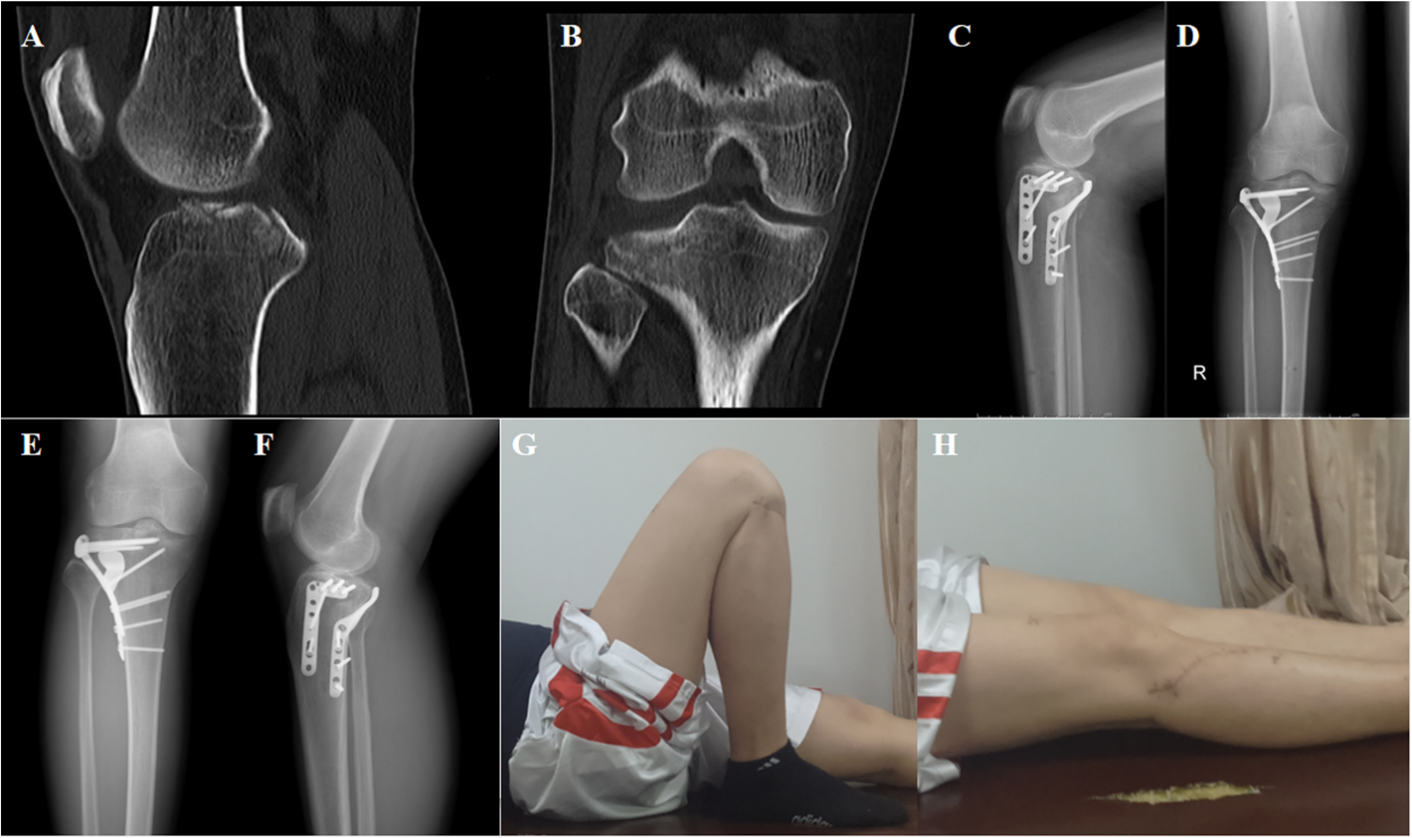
A 39-year-old man who sustained a right posterolateral tibial plateau fracture after a falling injury (Schatzker type II). A, B Preoperative computed tomography (CT), showing the posterolateral tibial plateau fracture. C, D Postoperative radiographs, showing the RSP fixation. E, F Follow-up radiographs after 1 year, showing complete union of fracture. G, H The clinical outcome was excellent
